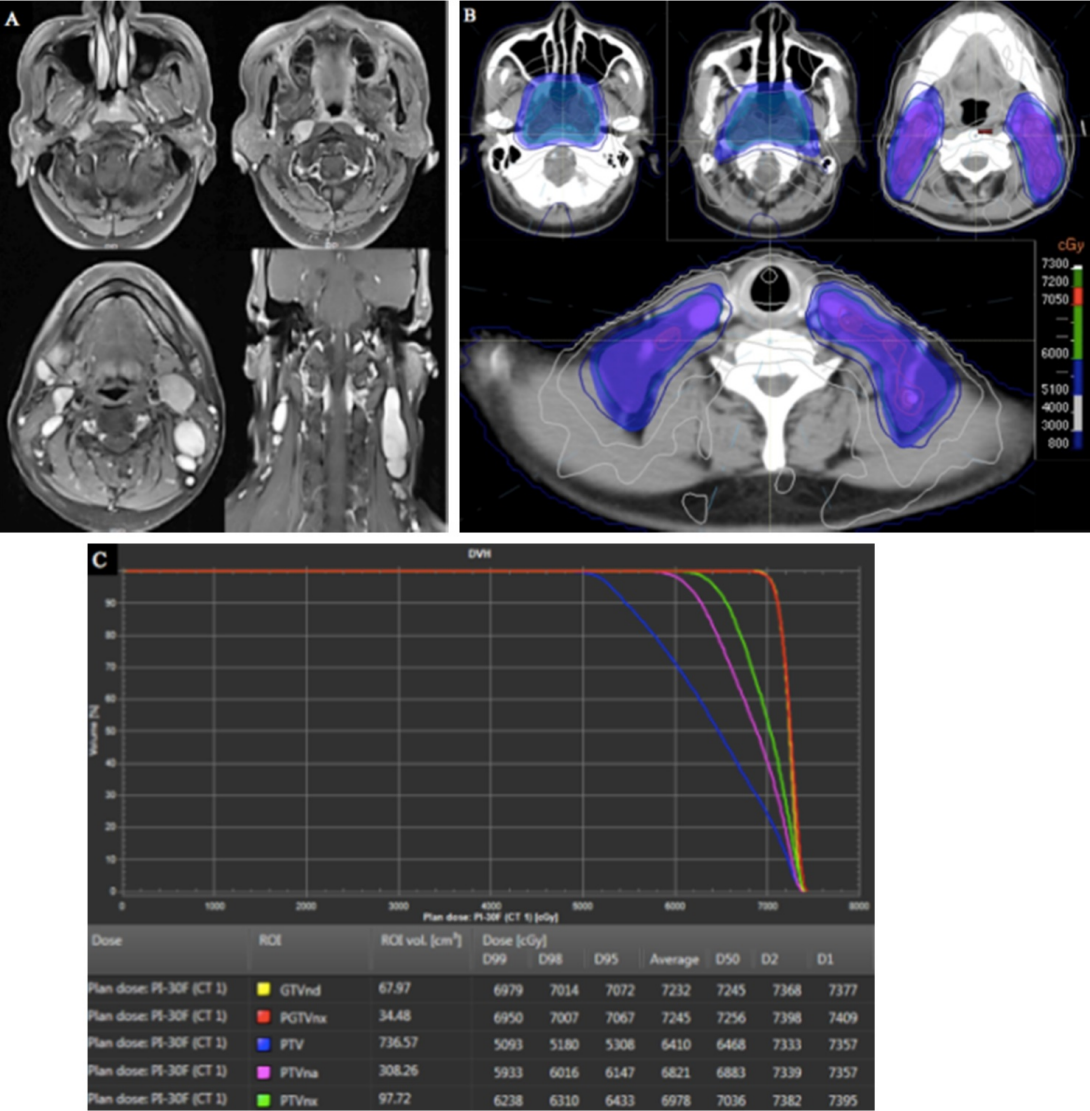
Target volumes and IMRT planning in a patient with stage T2N3M0 NPC. (A) MRI of the patient; (B) different risk target volumes and dose distributions; (C) DVH of IMRT. Abbreviations: IMRT: intensity-modulated radiotherapy; NPC: nasopharyngeal cancer; MRI, magnetic resonance imaging; DVH: dose-volume histogram.
